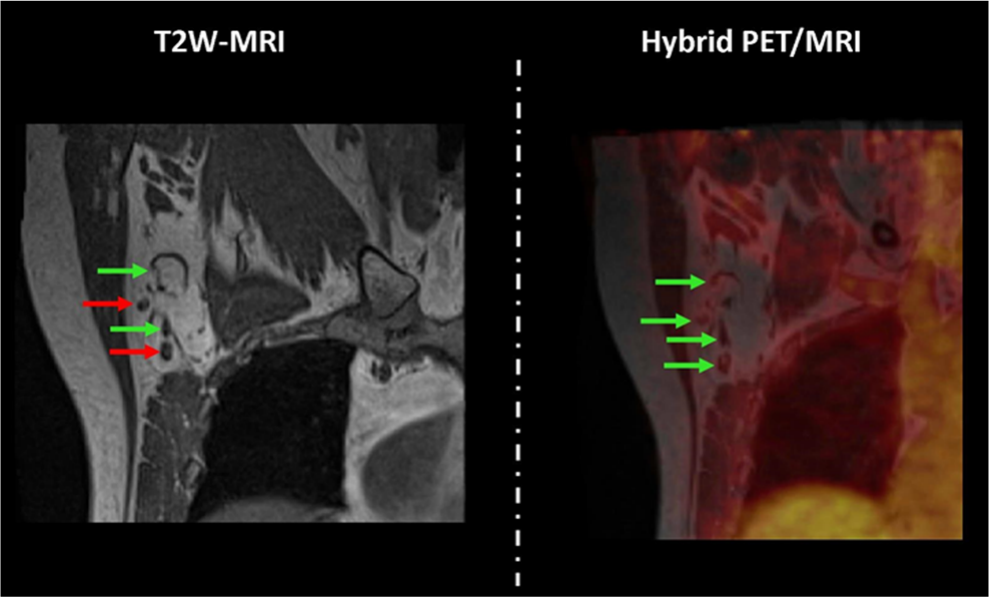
Example of a patient with a 31-mm invasive carcinoma of no special type in her right breast. A coronal T2-weighted MR image (*left*) of the right axilla demonstrates two (*red arrows*) of four suspicious nodes. A coronal dedicated axillary hybrid PET/MRI image (*right*) demonstrates no FDG-uptake in any of the four nodes (*green arrows*), which resulted in four negative nodes on dedicated axillary hybrid PET/MRI
